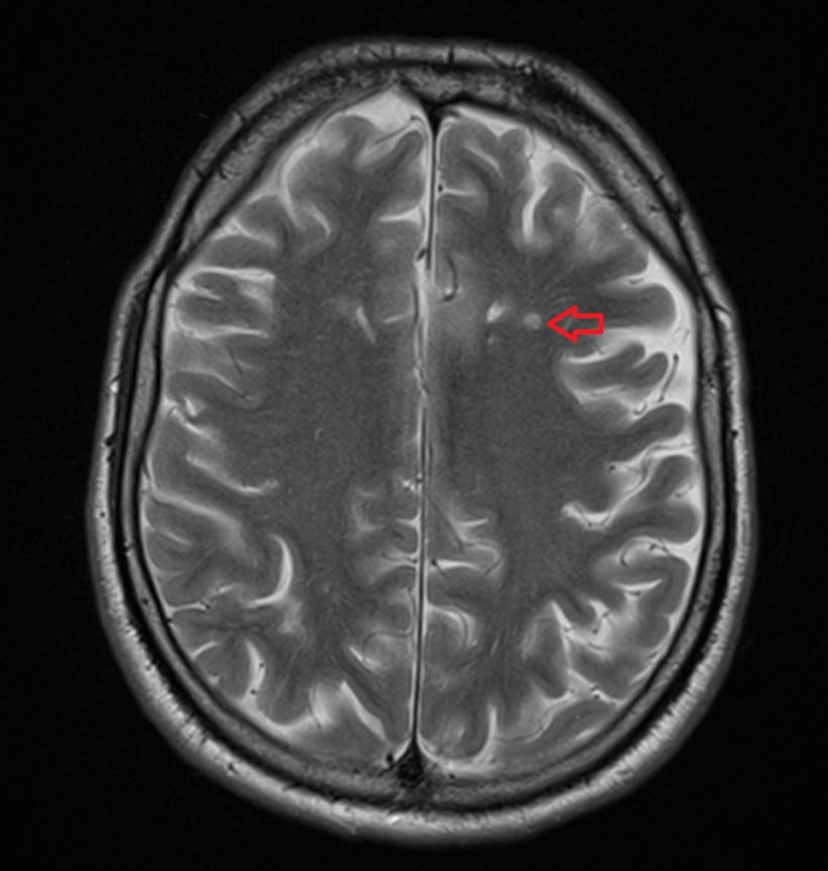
The only ischemic lesion inside the vascular territory of the probe occurred in the left frontal lobe of patient 1 (T2-weighted image of brain MR scan). The patient suffered from aneurysmal subarachnoid hemorrhage with subsequent severe vasospasm. The lesion resembles a small demarcated lacunar infarct (arrow). The most likely cause of ischemia in this patient was either vasospasm or an embolic lesion secondary to an angiographic intervention. However, it remains unclear, whether it was related to the introduction of the probe itself or represents a true ischemic lesion
